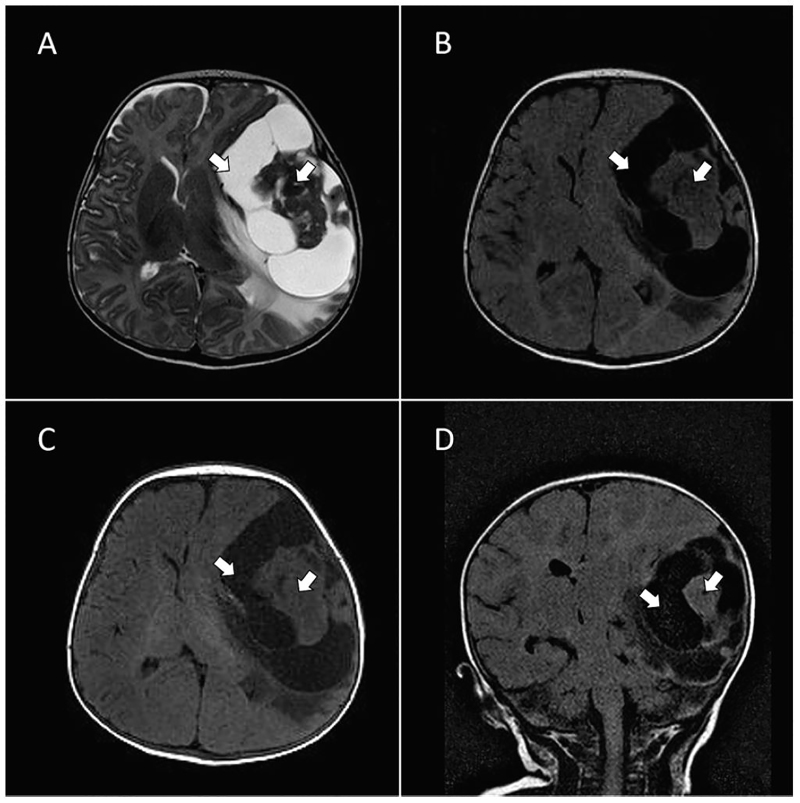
MRI revealing an 8.6cm × 7.9cm × 5.1cm semi-cysticsemi-solid mass (arrows) with epicenter in the left temporal region. T2-weighted axial view (A). T2-weighted with flair axial view (B). T1-weighted axial view (C). T2-weighed with flair coronal view (D). *MRI,* magnetic resonance imaging
